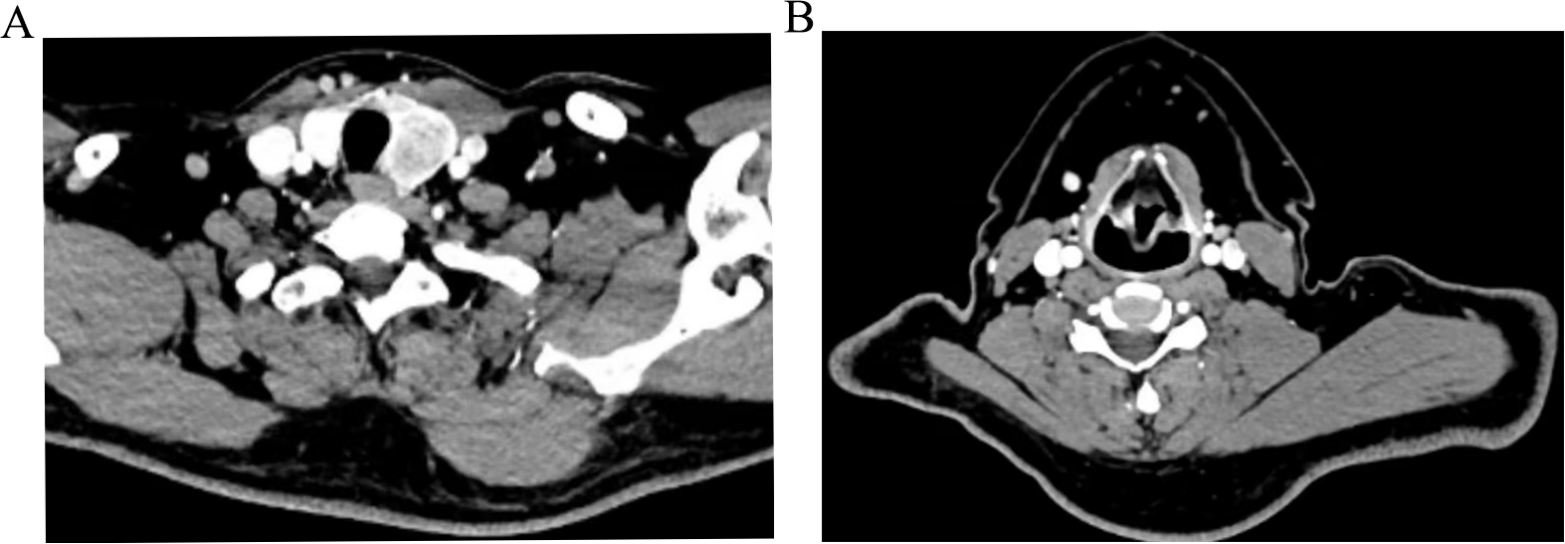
Preoperative CT images of the left thyroid (A), metastatic lymph nodes in the left neck region III (B).
